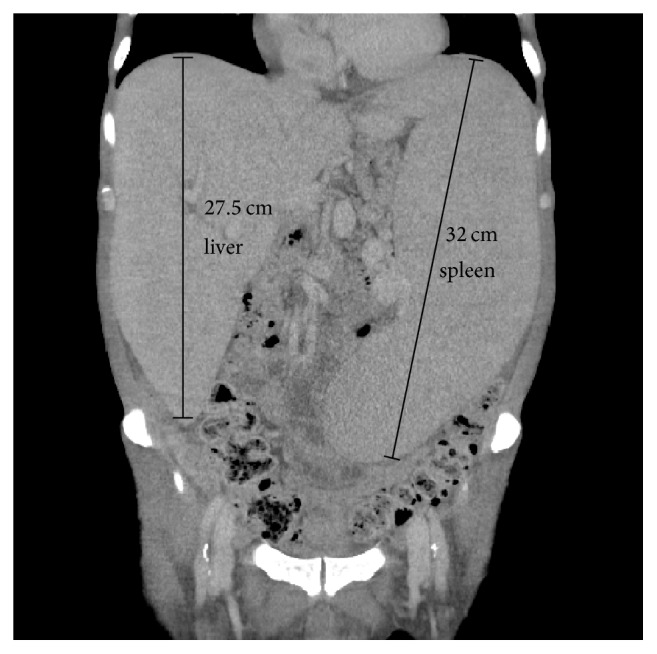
Preoperative CT demonstrating severe hepatosplenomegaly.
